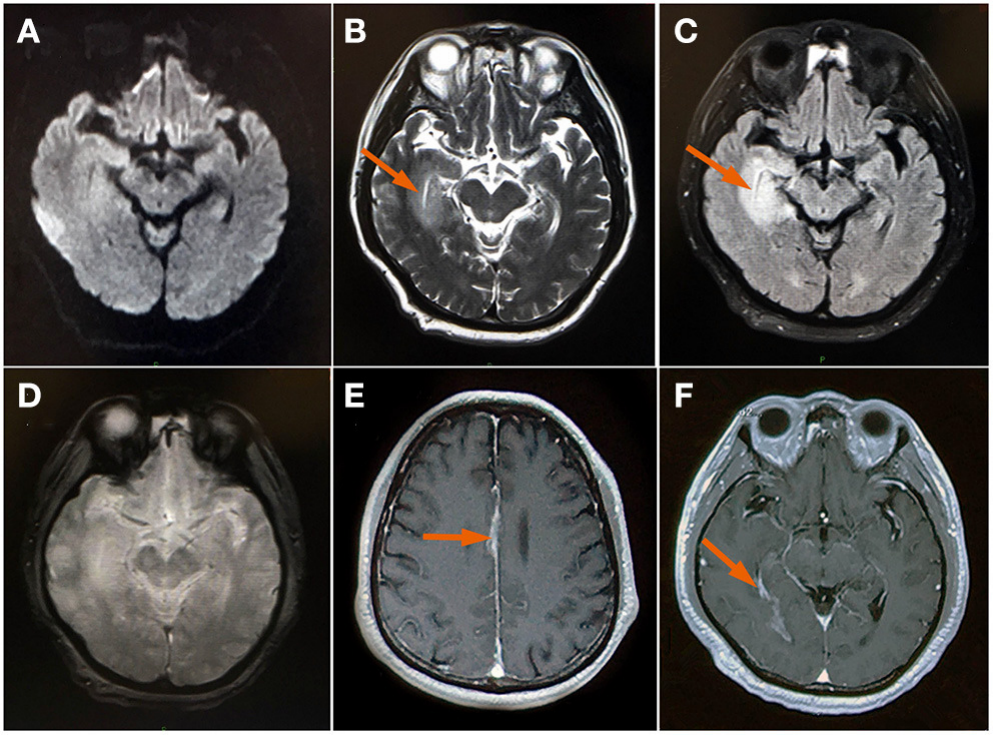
MRI brain (A) Axial DWI and (D) GRE sequence showed insignificant findings; (B) axial T2WI showed bright signal axial (arrow); (C) FLAIR image showed an edematous swollen temporal lobe (arrow); (E,F) Contrast-enhanced images demonstrated a fine linear enhancement of cerebral falx and bilateral choroid (arrow). DWI, diffusion-weighted imaging; FLAIR, fluid-attenuated inversion-recovery; GRE (gradient echo); T2WI, T2 weighted image.
